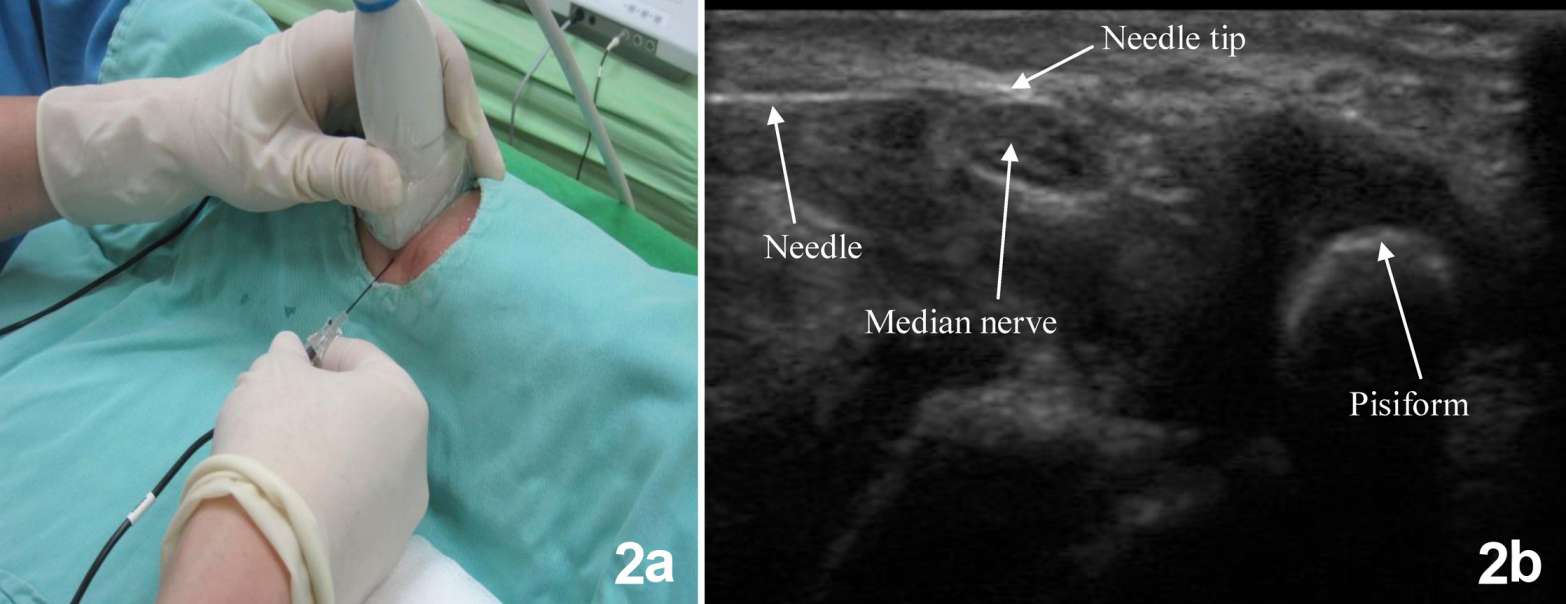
a: Positioning of the ultrasonographic transducer and radiofrequency needle. b: Real-time imaging of the ultrasound-guided needle insertion.
